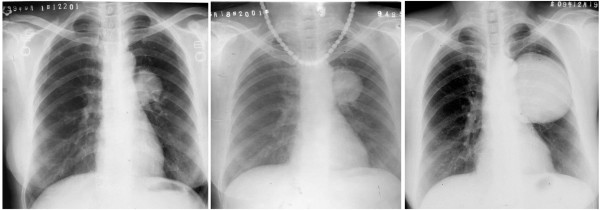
Chest radiograph of Case 1 obtained from 1999 to 2009. Chest radiograph of the first patient obtained in 2009 showing a gradual enlargement in the size of the nodule from its size in 1999.
